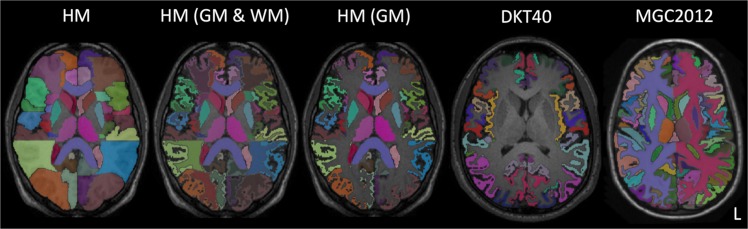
Axial cross-sections showing labels overlaid on the T1-weighted MRI of a sample subject from each of the atlas databases. Left to right: the unmodified HM atlas database, the HM atlas database with both grey-matter and white-matter sub-segmentations, the grey-matter masked HM atlas database, the DKT40 atlas database and the MGC2012 atlas database. Label colours are randomly assigned.
